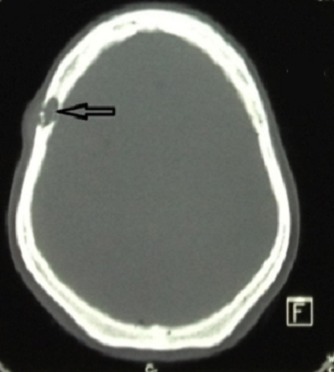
Axial CT scan slide, showing the metastatic frontal bone lytic lesion (arrow)
